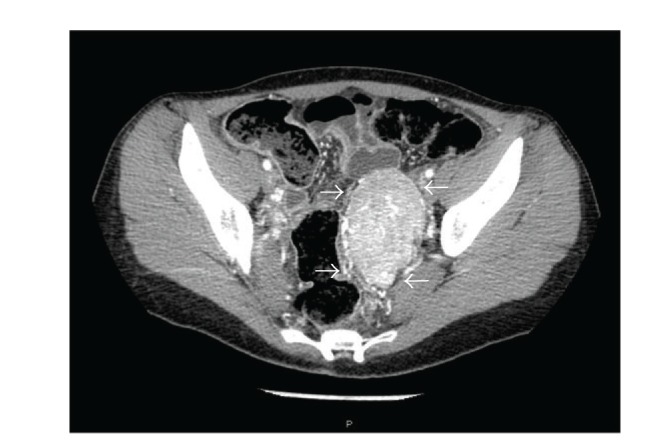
Enhanced pelvic CT scan showing a large mass in the left adnex site.
